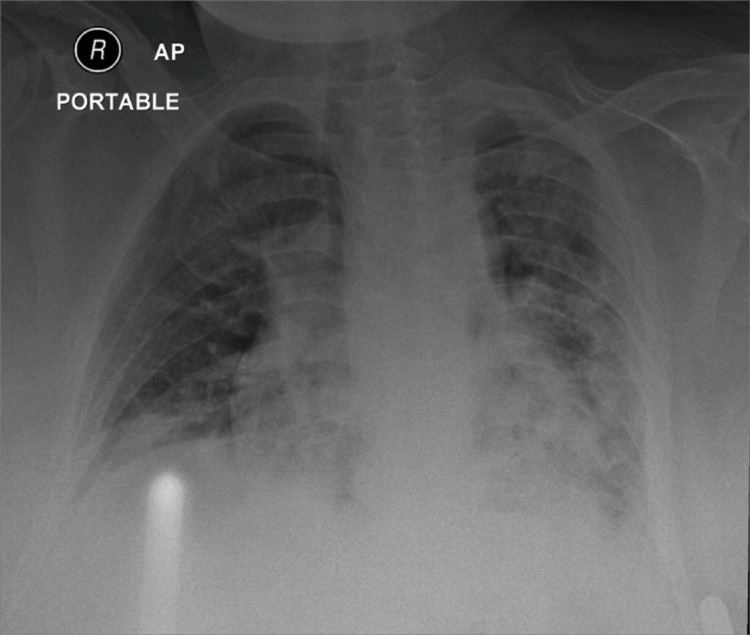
Chest X-ray revealing bilateral basal lung infiltration in the COVID-19 patient COVID-19, coronavirus disease 2019
